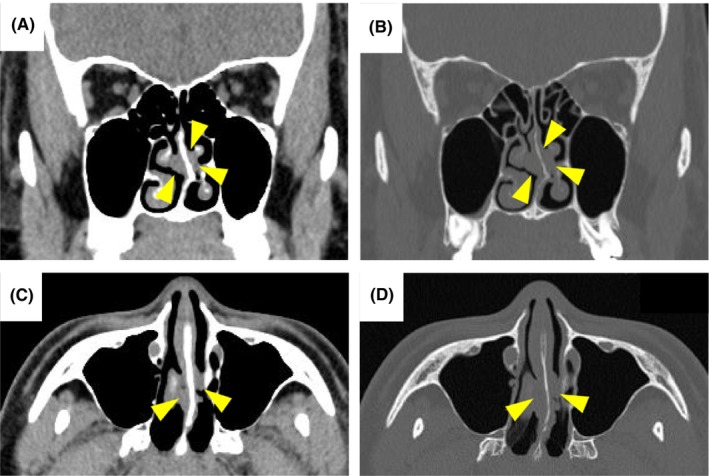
Computed tomography findings of the coronal view (A), coronal view with bone window (B), axial view (C), and axial view with bone window (D) are shown. A mass is visible in the nasal septum (arrowhead). No obscuration of the perpendicular plate of the ethmoid bone is present.
